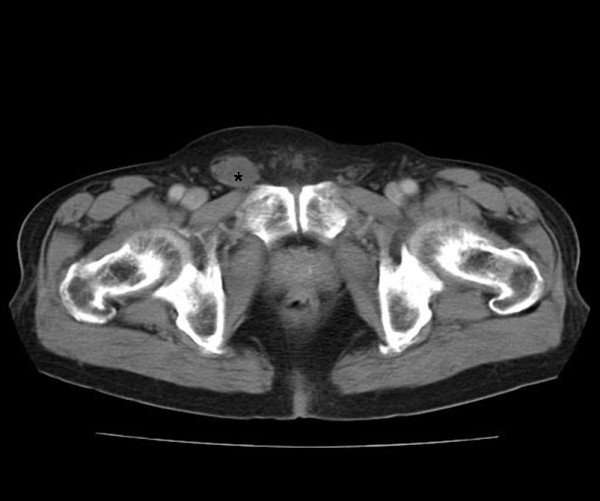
Contrast-enhanced CT scan on the pelvis. It reveals an oval shaped, low attenuation mass (asterisk), measuring 5.0x2.5x2.1 cm, and showing a well demarcated smooth margin in the right inguinal area.
